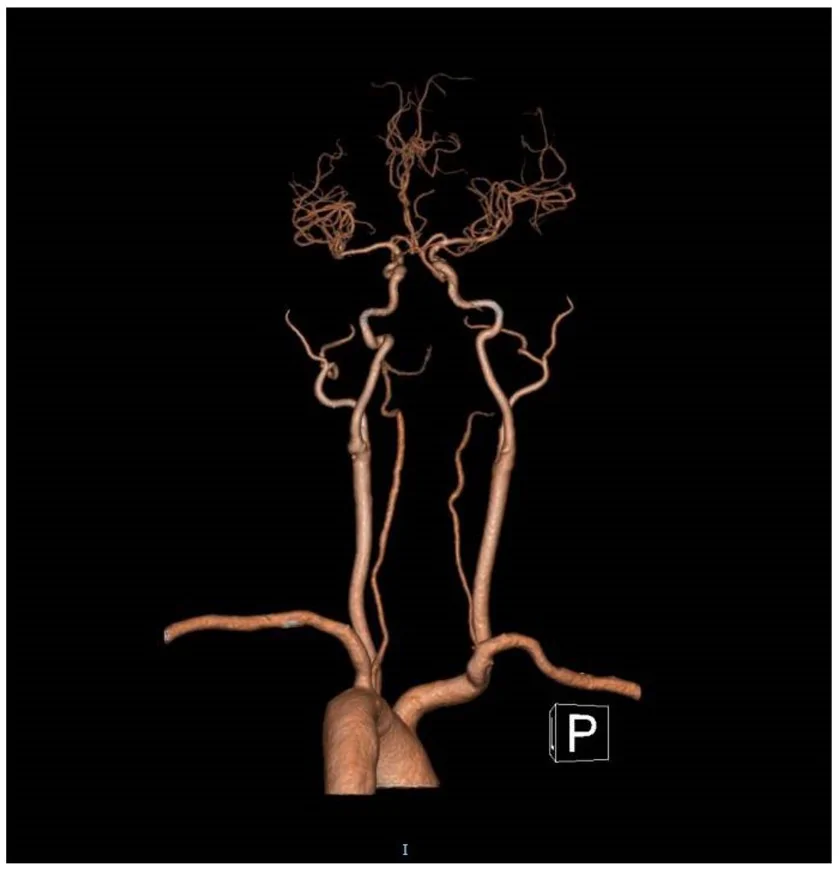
The computed tomography angiography (CTA) of the head on January 12, 2023. Both sites of bilateral vertebrobasilar intersection were not visible.
